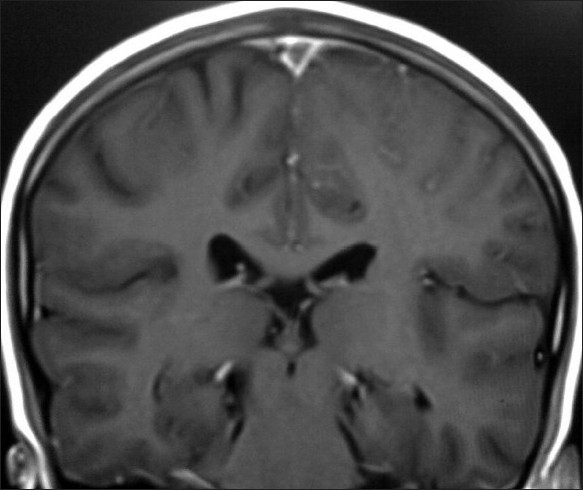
Coronal T1-weighted postcontrast MR image showing empty delta sign due to superior sagittal sinus thrombosis
